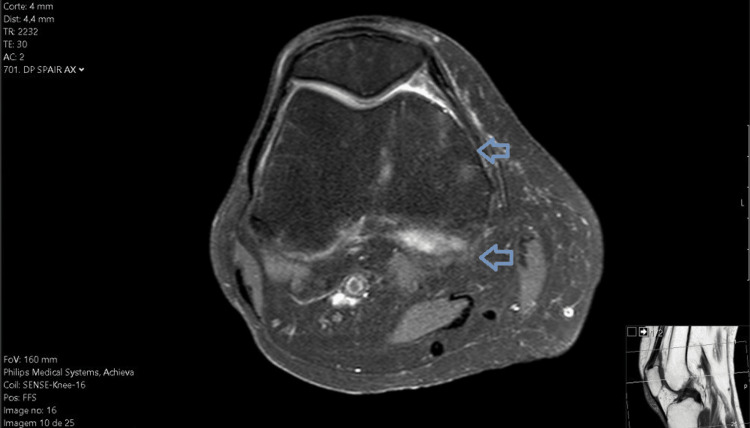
MRI of the right knee in the axial plane (re-evaluation).
